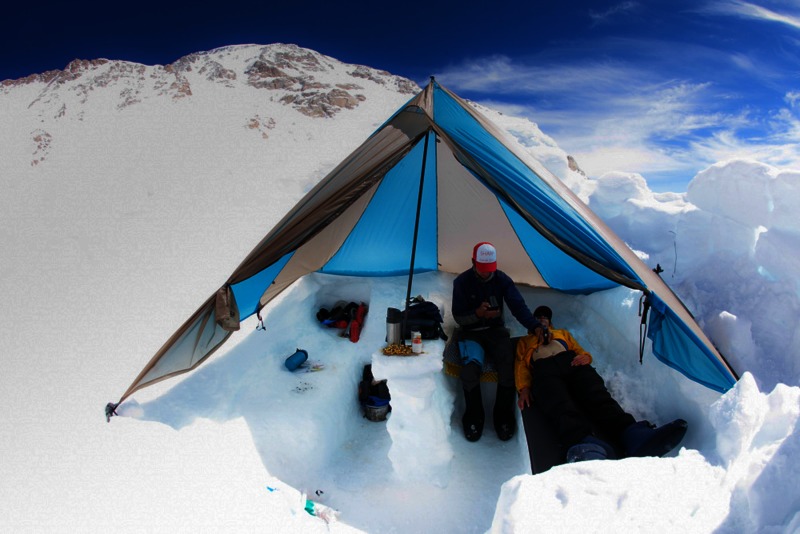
Study enrollment and ultrasound data acquisition site
